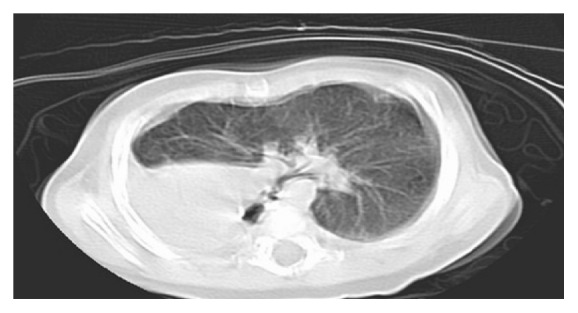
CT chest revealed right lung agenesis, dextrocardia, compression of the distal trachea, and proximal left bronchus beneath the aorta.
